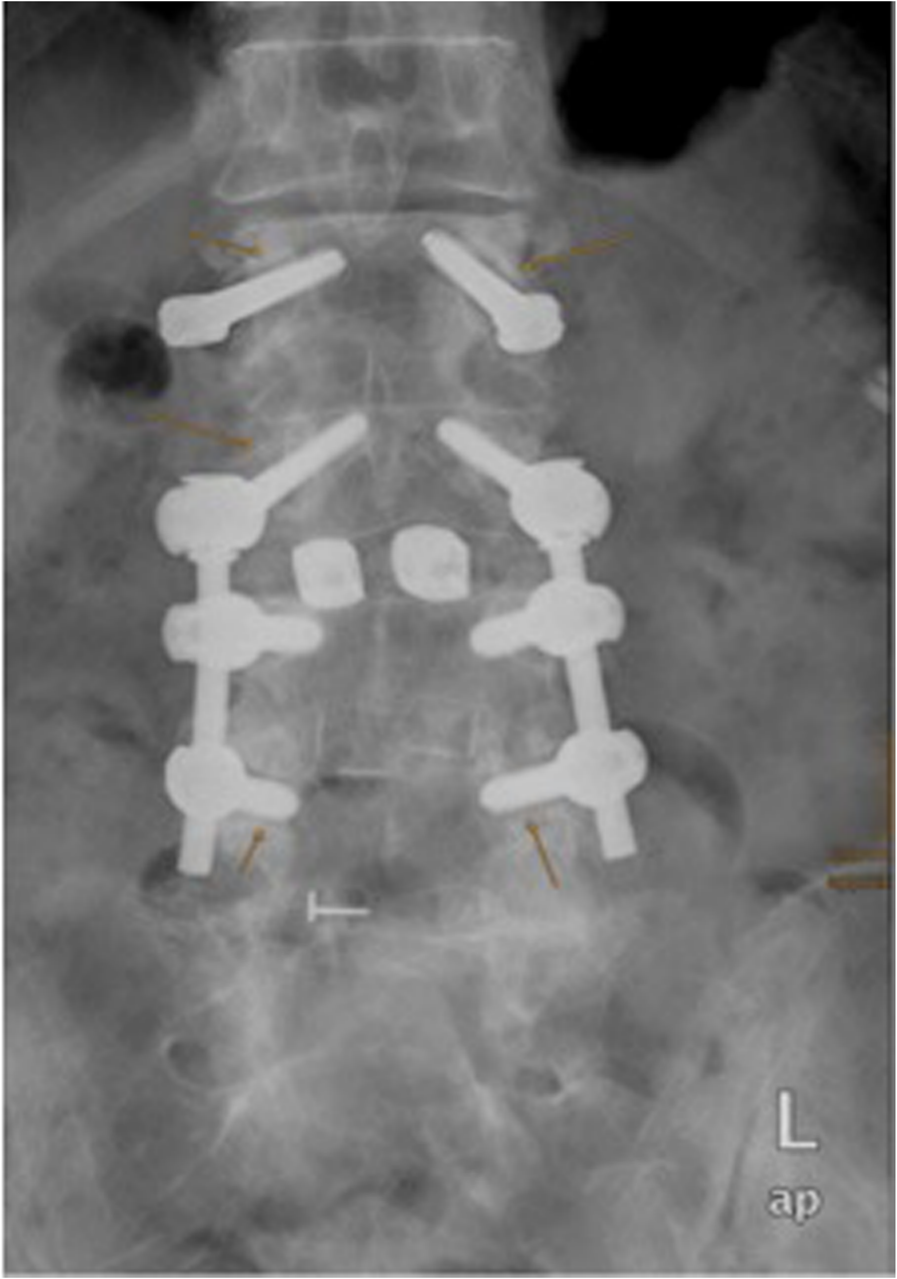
Radiograph of the lumbar spine in ap-view. Slight signs of implantat loosening within L2,L3 and L5 (marked with a yellow arrow)
